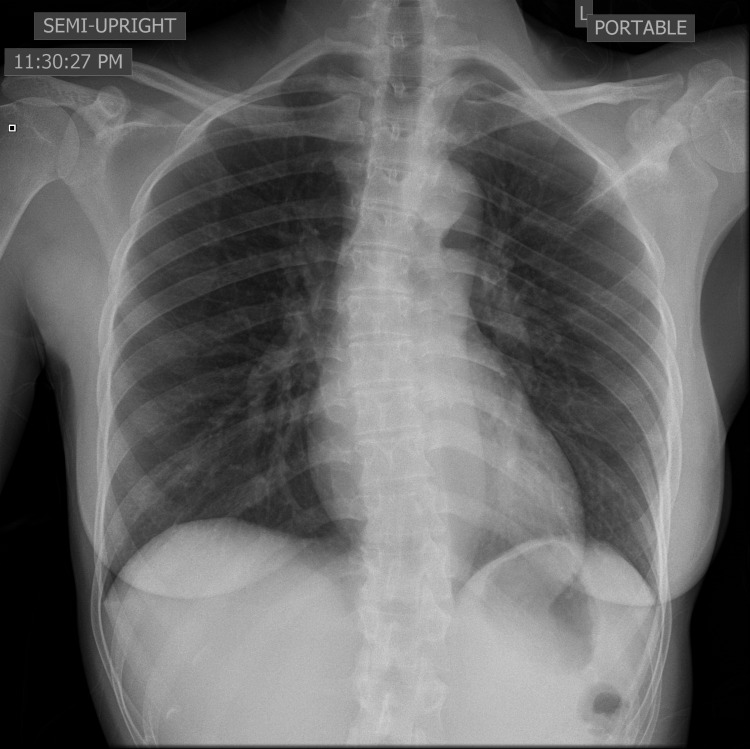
No acute cardiopulmonary changes on the chest X-ray
